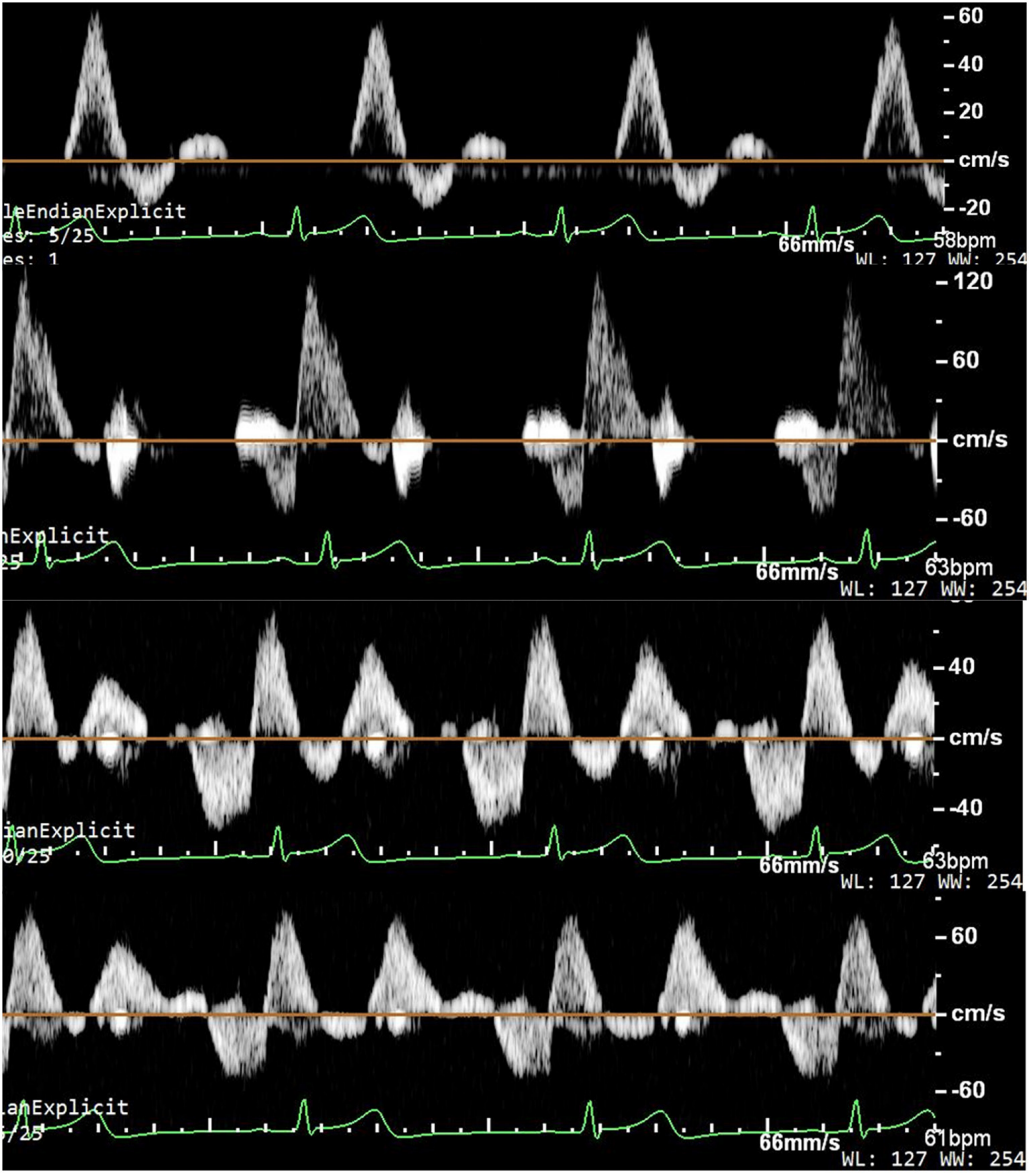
Ultrasound pictures and Doppler spectrum of the anterior tibial artery at baseline, during EECP-3, EECP-1, and EECP-2.
